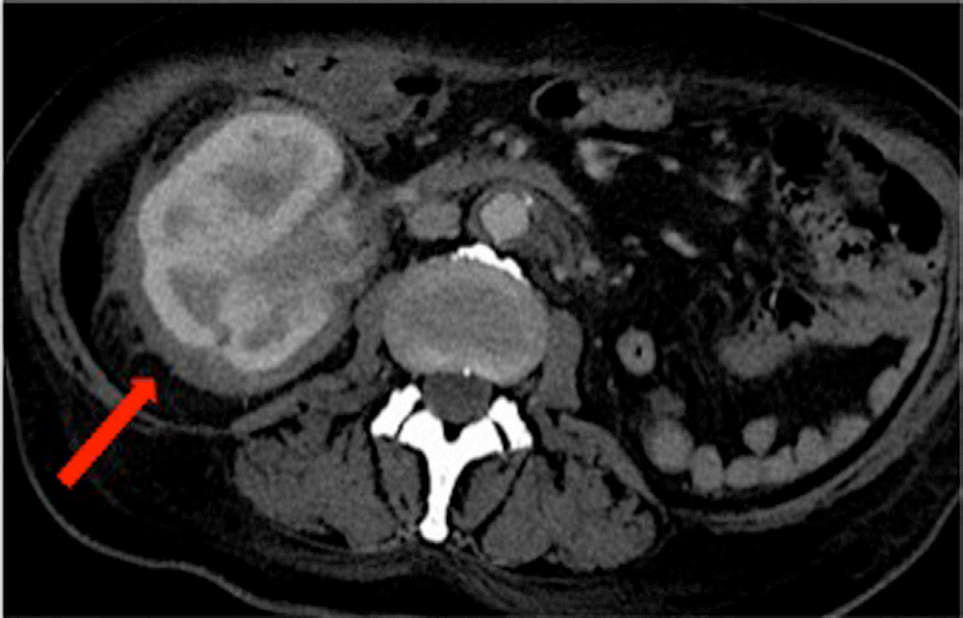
Computed tomography scan of the abdomen revealing solid tissue surrounding the right kidney (commonly referred to as “hairy kidney”) [*red arrow*] and circumferential peri-aortic sheathing (“coated aorta”).
